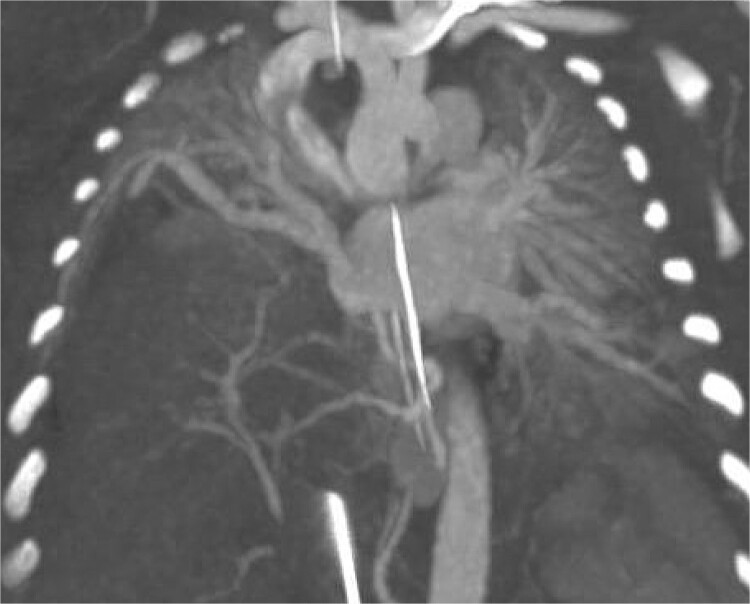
CT arteriography: coronal slice demonstrating anatomy of pulmonary veins with two left sided pulmonary veins draining into the left atrium and the single right sided upper pulmonary vein also draining into the left atrium.
